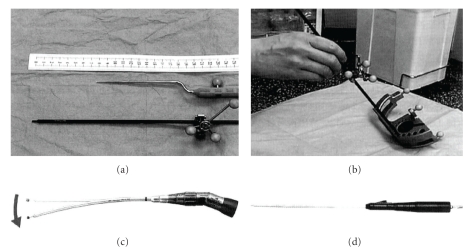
An original long navigation pointer (a) registered by the Universal Instrument Integration system ((b): VectorVision: BrainLAB Co., Ltd.). Long and slim instruments we used for this operation, highspeed drill ((c): Primado: Nakanishi Co., Ltd.) and ultrasonic aspirator ((d): SONOPET: M&M Co., Ltd.).
